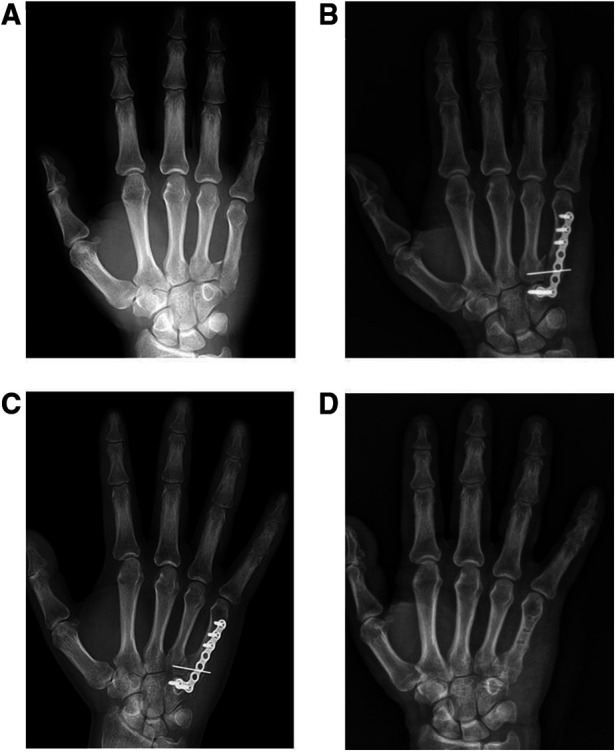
The treatment process of a patient with fracture-dislocation of the fifth carpometacarpal joint of the right hand. (A) The preoperative anteroposterior x-ray. (B) The postoperative anteroposterior x-ray the day after internal fixation surgery. (C) The postoperative anteroposterior x-ray 10 weeks after internal fixation surgery. (D) The postoperative anteroposterior x-ray the day after internal fixation removal surgery.
